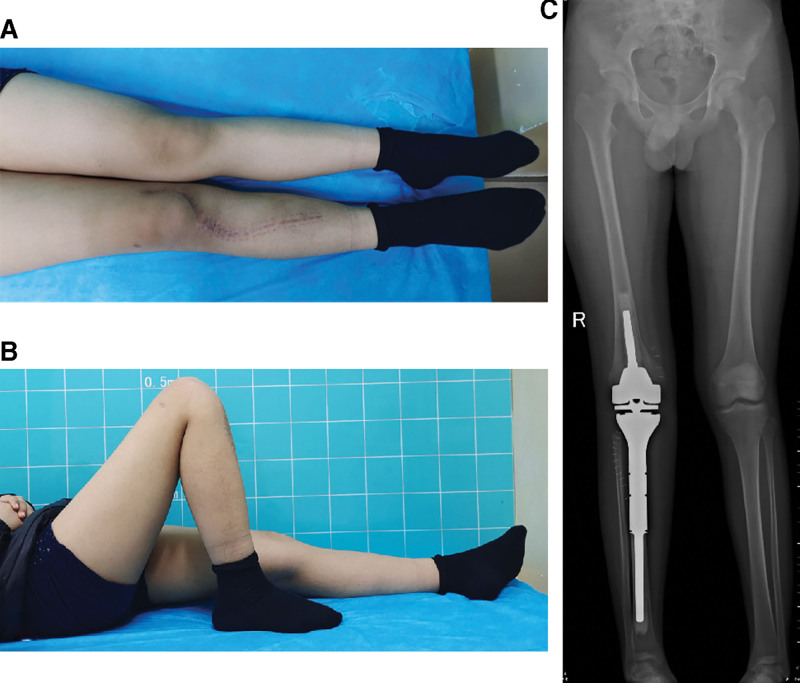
The incision healed well after operation (A). The function and activity of the right knee joint are basically normal (B). The postoperative plain radiograph showed good prothesis location without loosening; the small head of the right fibula was absent, and the length of both lower limbs was basically the same (C).
